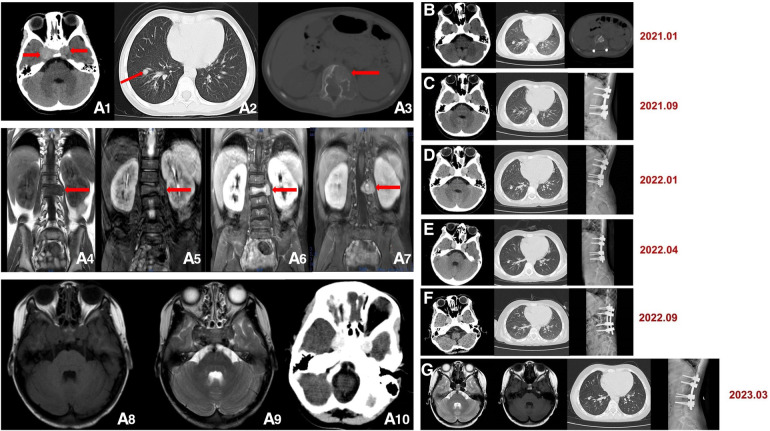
(A1–10) Initial computed tomography (CT) and magnetic resonance imaging (MRI) scans. (A1) Head CT shows bilateral circular nodules of soft tissue near the cavernous sinus (red arrow), approximately 15 × 13 mm (left) and 20 × 17 mm (right). (A2) Lung CT shows multiple nodules in the lungs, with one of the nodules in the right lower lobe and the rest in the basal segment, approximately 7 mm in diameter (red arrow). (A3) Lumbar CT shows bone destruction and soft tissue tumours of the L2 vertebral body and left side of the accessory area (red arrow). Lumbar MRI shows an area of hyposignal intensity in (A4) T1 imaging and slight hyposignal intensity in (A5) T2 imaging (red arrow). (A6) and (A7) Lumbar-enhanced MRI showed flattening of the L2 vertebral body and bone destruction in the left part of the vertebral body and the vertebral arch adnexa. A 25 × 25 mm local irregular soft tissue lesion was found (red arrow). (A8) and (A9) Head MRI. (A10) Enhanced head CT. (B–G) Follow-up images after surgery. Head CT, lung CT and head MRI show that no significant change occurred in the lesions. Lumbar CT and x-ray show postoperative changes.
